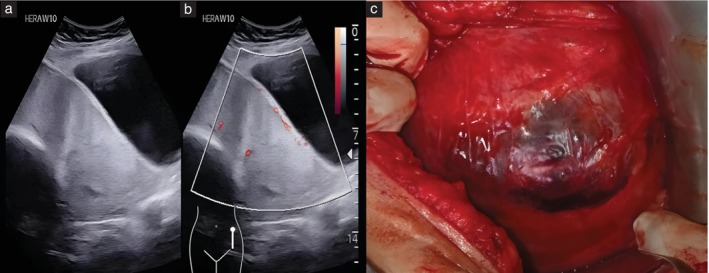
(a,b) Ultrasound images in a patient with two previous Cesarean deliveries and anterior placenta previa. (a) On grayscale ultrasound, there was significant myometrial thinning at the lower uterine segment, with loss of the retroplacental clear zone. (b) Color Doppler ultrasound revealed the absence of uteroplacental vascular remodeling and serosal hypervascularity features. (c) The intraoperative image demonstrates an obvious placental bulge, with the placenta visible underneath the scar and absence of hypervascularity on the serosal surface. The placenta separated spontaneously from the uterine wall, confirming the diagnosis of uterine‐scar dehiscence with an underlying non‐adherent placenta.
